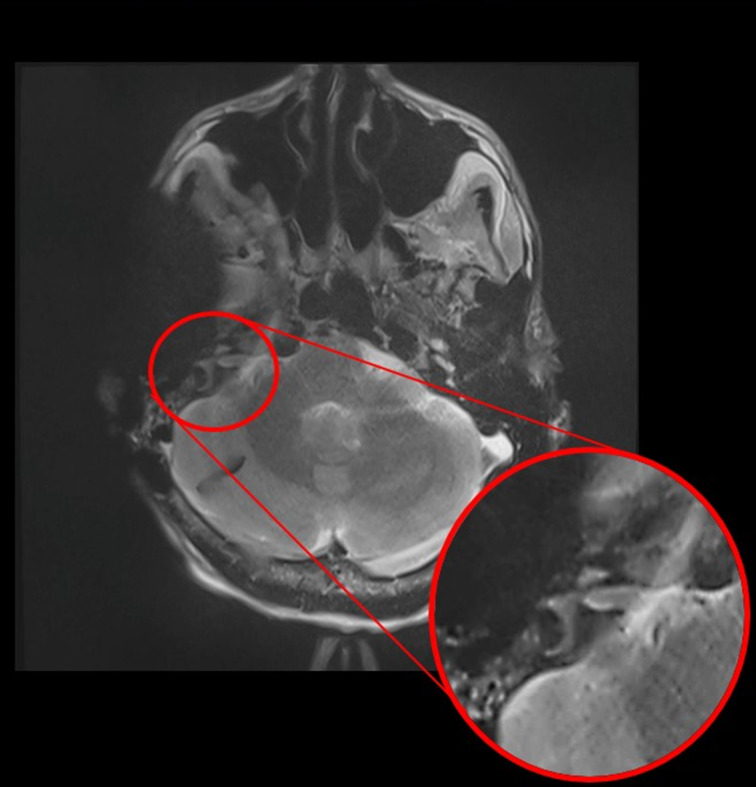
Position 1 T2-weighted axial magnetic resonance imaging scan with slice-encoding metal artifact correction view-angle tilting metal artifact reduction technique sequences. Visualisation of the brain adjacent to the artefact of the bone-conduction hearing implant 602 device with a zoom-in of the area of interest: the cerebellopontine angle and internal auditory canal are fully visible.
